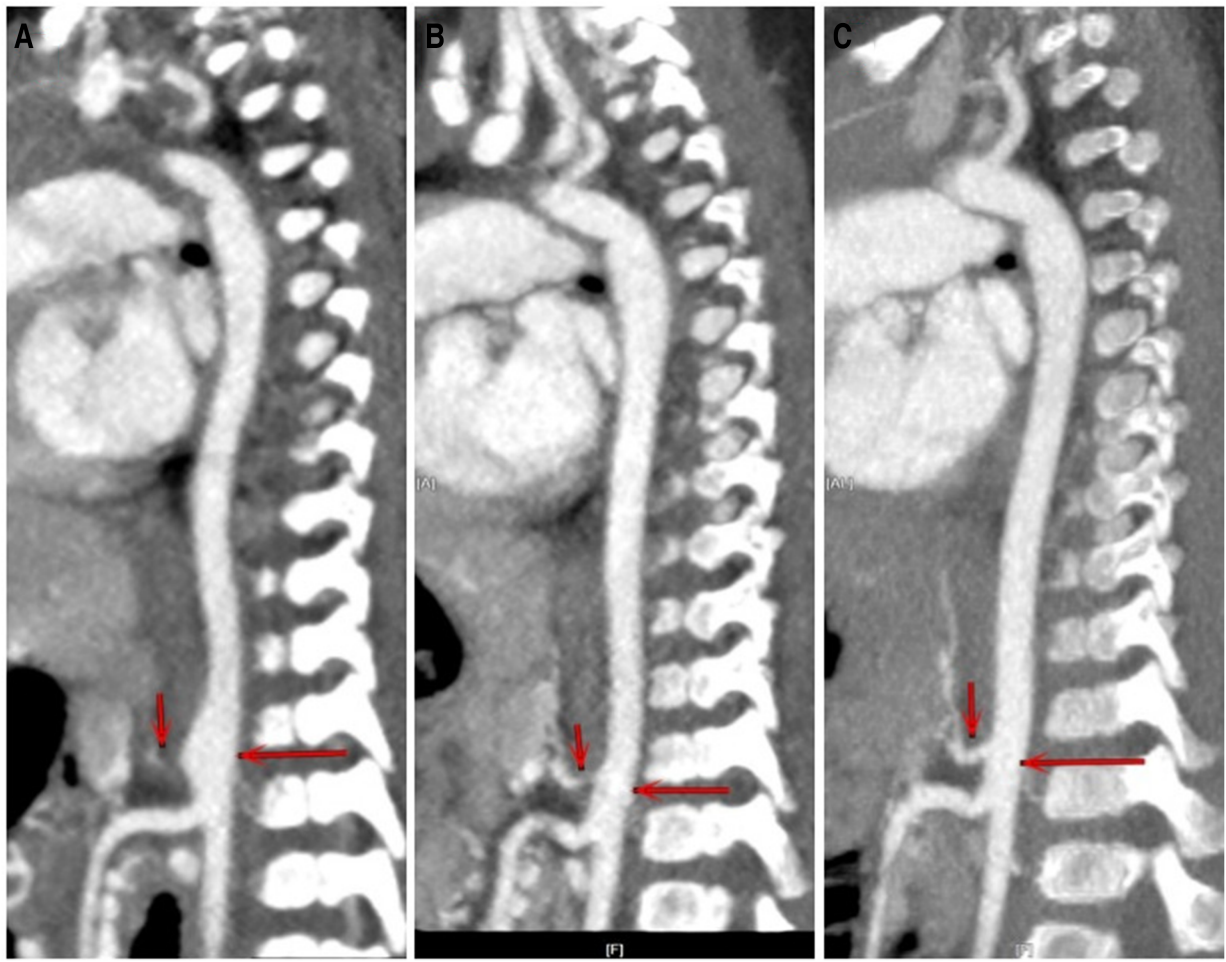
CTA of case 1 at different stages. (A) At first clinic visit. (B) After three and a half months of treatment. (C) After 1 year of treatment. The involved aorta improved to a great extent after treatment.
